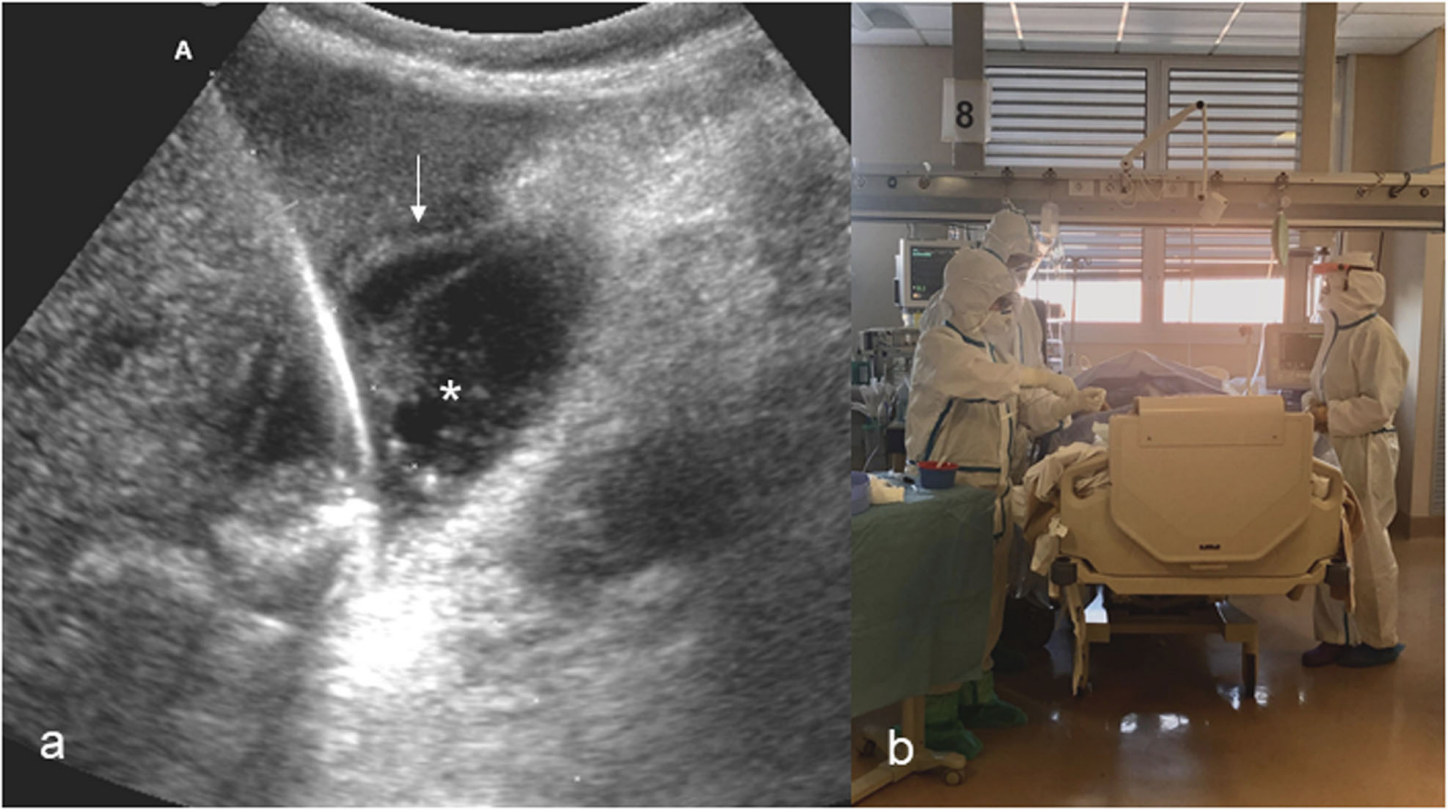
Percutaneous transhepatic ultrasound-guided cholecystostomy through an intercostal approach in a coronavirus disease 2019, COVID-19, patient with acute cholecystitis. The guidewire (A), the “railway sign” of the gallbladder wall (arrow), and the presence of sludge and microlithiasis (asterisk) are shown in a. The interventional radiology team performing percutaneous gallbladder drainage at the bedside in intensive care unit is shown in b
